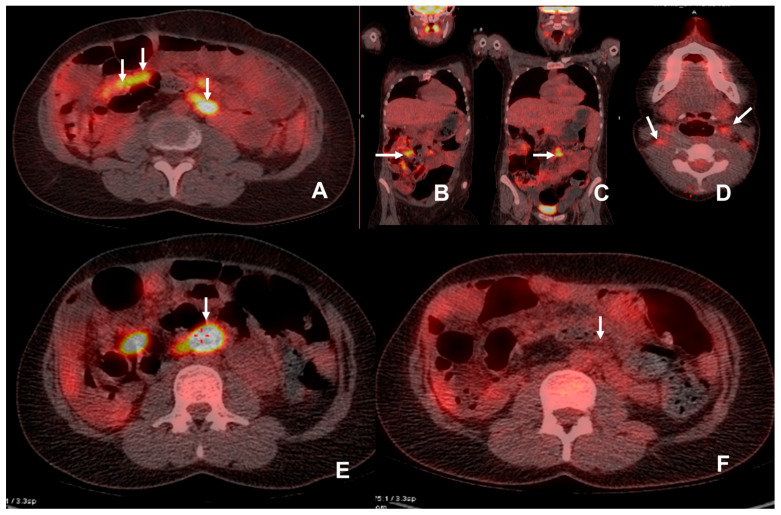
The patient was admitted with an elevated amylase level 6 years post multivisceral transplant. CT demonstrated increased prominence of mesenteric lymph nodes and there was a clinical suspicion of PTLD. ^18^F-FDG PET/CT demonstrated multiple FDG avid (SUVmax 12.6) mesenteric lymph nodes (white arrows)(A–C). Biopsy demonstrated a monomorphic plasma cell infiltration in keeping with PTLD. Anti-rejection/immunosuppressant therapy was reduced in an attempt to control the PTLD. Follow-up ^18^F-FDG PET/CT examination was performed at 2.5 months (D,E) due to an inability to biopsy the deep-seated lymph node in a difficult abdomen with significant thrombocytopenia. This showed a marked increase in SUV (SUVmax 23.7) and the size of the mesenteric lymph nodes, a large FDG avid para-aortic lymph node (white arrow with adjacent red crosshairs, image (E)) and additional involvement of cervical lymph nodes (white arrows, image (D)) with SUVmax at 4.6, in keeping with progressive PTLD. Results of this examination were discussed at MDT and Rituximab was commenced. A further follow-up ^18^F-FDG PET/CT was performed 10 weeks after the initiation of Rituxiamb and demonstrated resolution with a significant drop in SUV (F) for the reference nodes.
